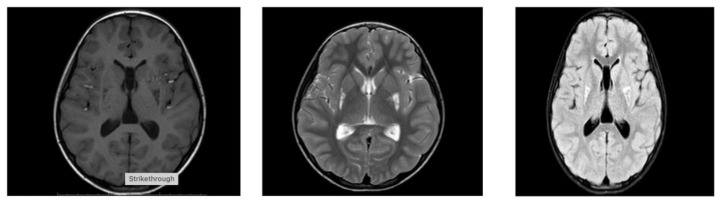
Hypointensity on T1 and hyperintensity on T2 and T2 FLAIR sections in the lower half of the bilateral lenticular nuclei.
